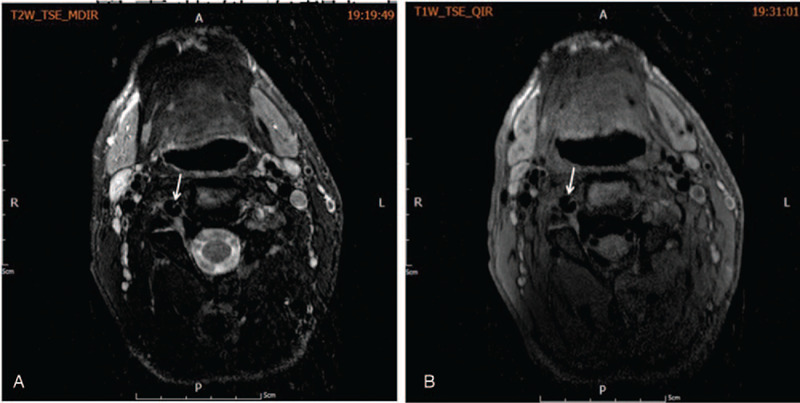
Magnetic resonance imaging (HR-MRI) findings. Irregular stenosis and intimal flap in the right VA (arrows) and occlusion of the left VA were observed. HR-MRI = high-resolution magnetic resonance imaging, VA = vertebral artery.
